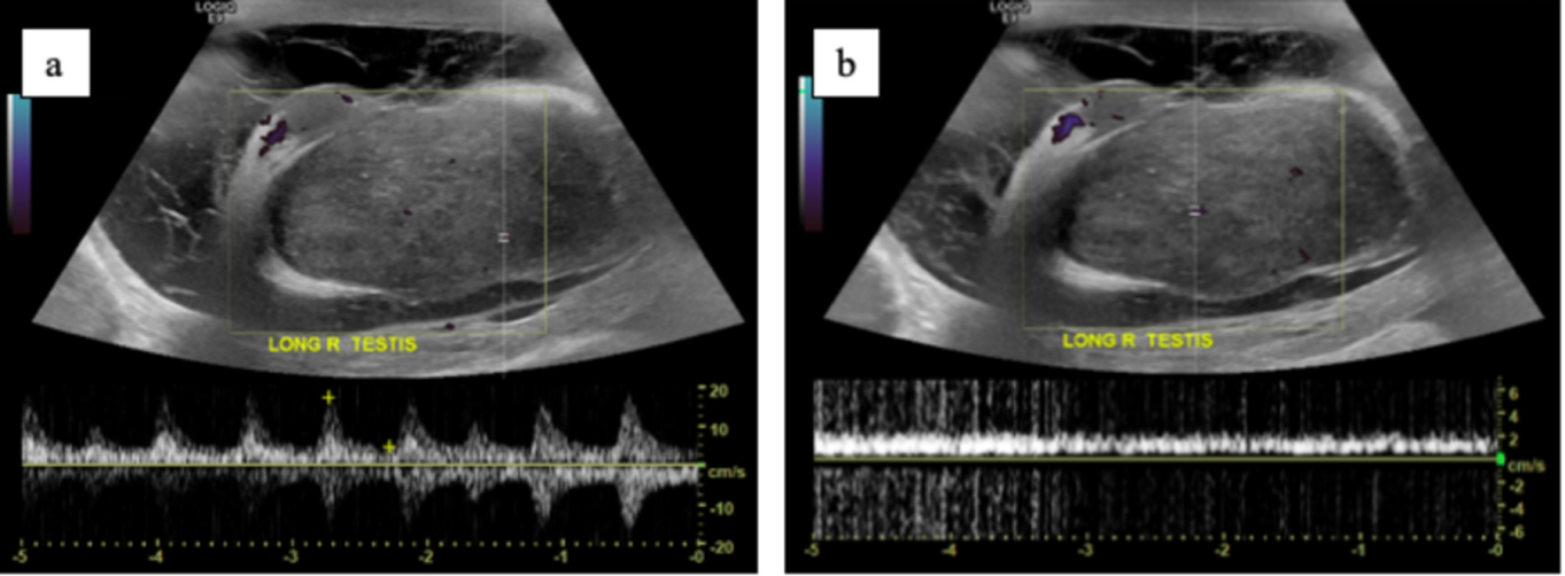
Spectral Doppler ultrasound of right testicle demonstrating normal arterial (a) and venous (b) flow.
